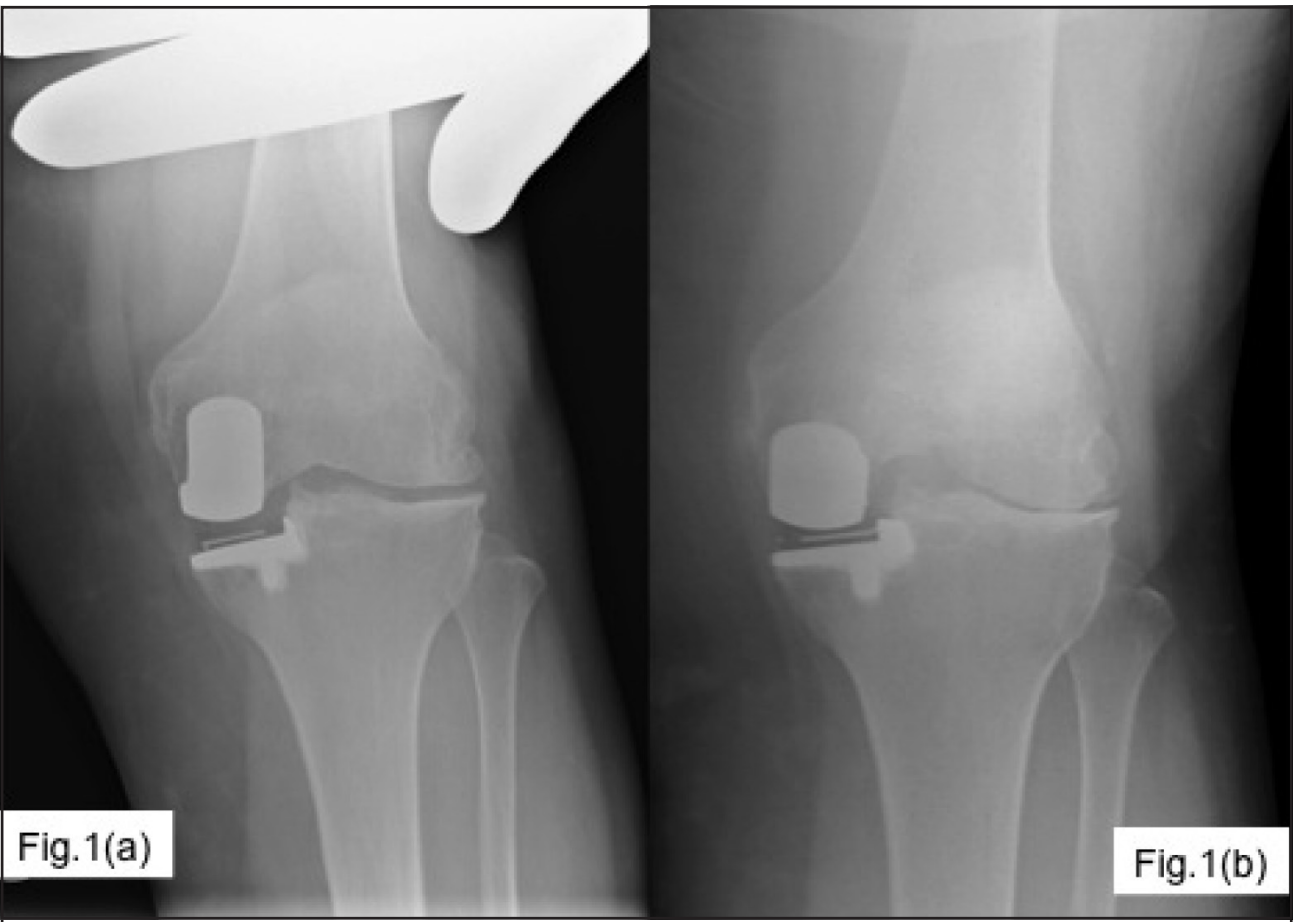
X-ray evaluation of a painful unicompartmental knee replacement. 1(a): AP valgus stress X-ray, 1(b): Lyon Schuss view.
